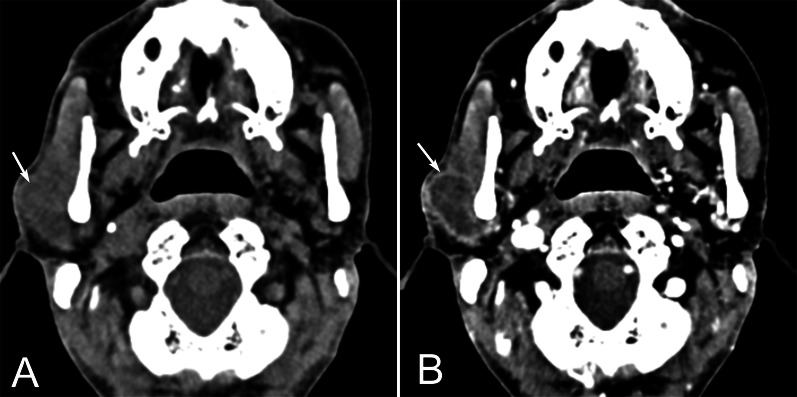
A 65 years old male with primary parotid SCC in the right parotid gland. Axial non-contrast CT (A) shows an ill-defined, lobulated and hypodense lesion in the superficial part of the right parotid gland (arrow). Contrast-enhanced CT (B) demonstrates moderate rim enhancement with severe central necrosis (arrow)
